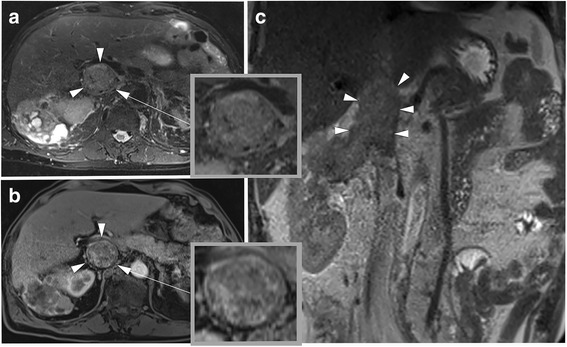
Images in a 55-year old man with a clear cell renal cell carcinoma (RCC) and an inferior vena cava (IVC) tumor thrombus with wall invasion. The RCC extends from the right kidney into the suprahepatic IVC. a axial fat-saturated T2-weighted image. b T1-weighted contrast-enhanced 3D GRE (VIBE) image (arterial phase) and (c), coronal T2-weighted HASTE image for anatomic reference. Note that the thrombus completely obstructs the lumen of the IVC and shows direct contact with the vessel wall (a, c). The contrast-enhanced image (b) demonstrates a heterogeneous enhancement of the tumor thrombus, and contact to, but no breach of the vessel wall, which makes IVC wall invasion likely. During extended nephrectomy, this thrombus was partly adherent the IVC and after extraction of the IVC thrombus, continuous suturing became necessary. VIBE = Volumetric interpolated breath-hold examination
